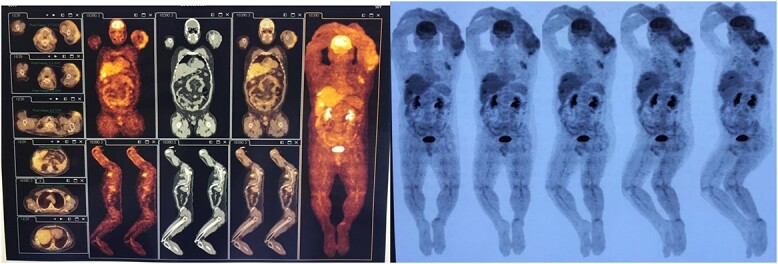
Positron emission tomography-computed tomography scan revealed hypermetabolic lesions. It shows local and distant metastases to the lymph nodes, liver and bone.
